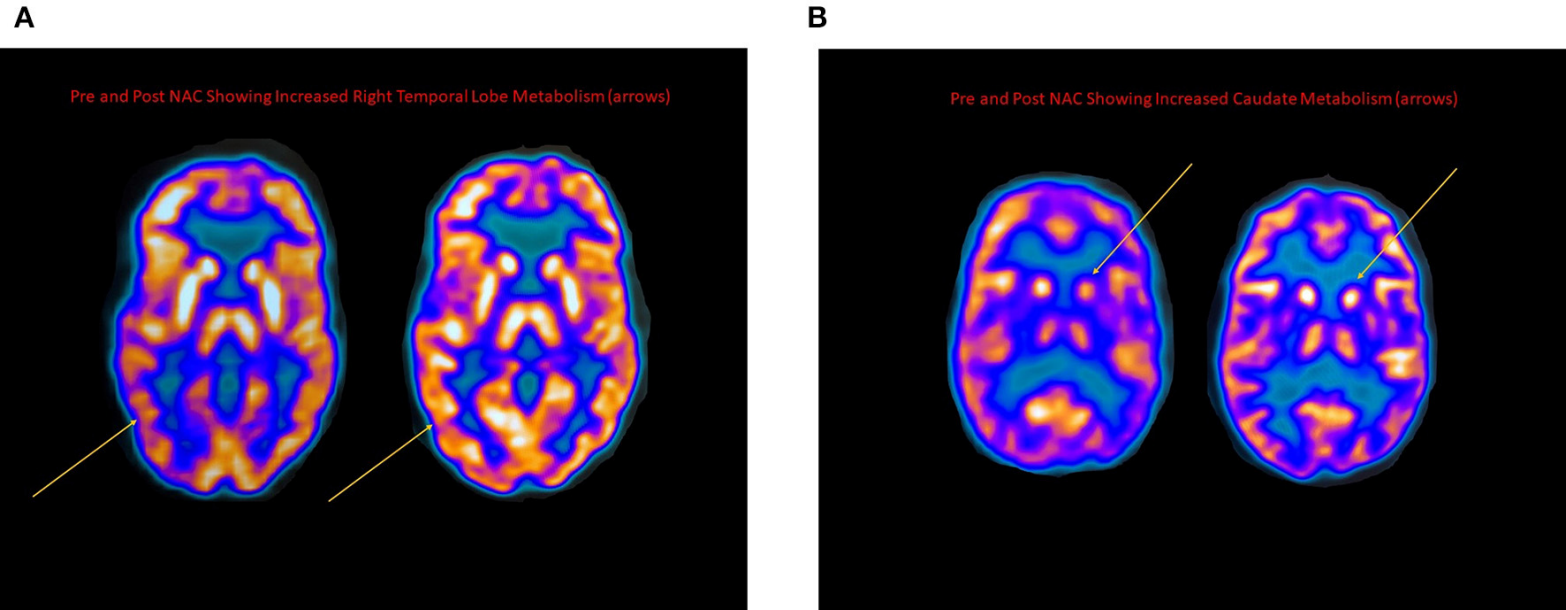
(A) FDG PET scans taken pre and post-NAC for an MS patient demonstrating marked improvement in the right temporal lobe cerebral glucose metabolism (arrows). (B) FDG PET scans taken pre and post-NAC for an MS patient demonstrating marked increase in caudate glucose metabolism (arrows).
